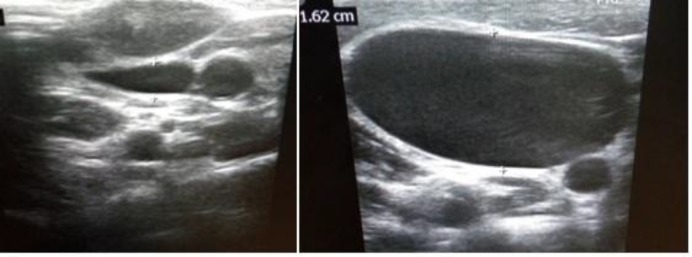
A- showing pre valsalva diameter of Right IJV on Ultrasound Neck. B- showing post valsalva diameter of Right IJV on Ultrasound Neck
